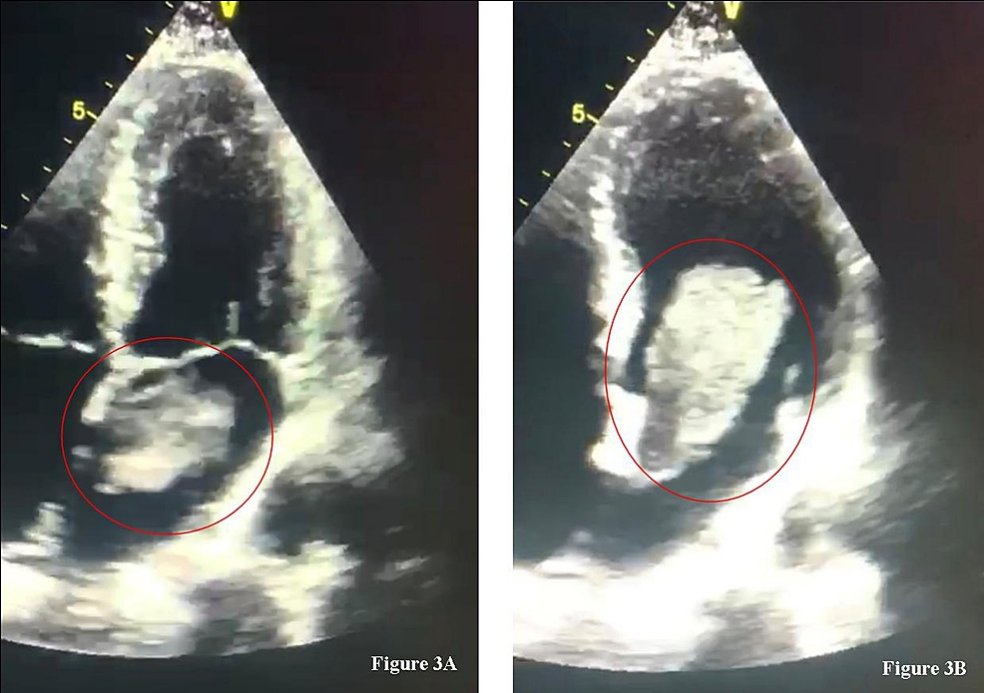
Echocardiographic findings Presence of a pedonculated mass in the left atrium (Figure 3 A) that penetrates the left ventricle during diastole (Figure 3 B)
